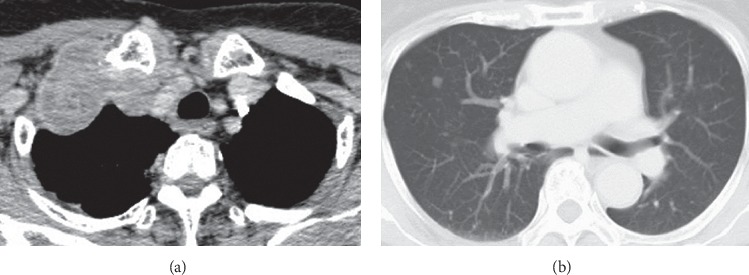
Images taken during the initial visit. Computed tomography revealed a large right-upper mediastinal tumor (a) and micropulmonary metastases (b).
